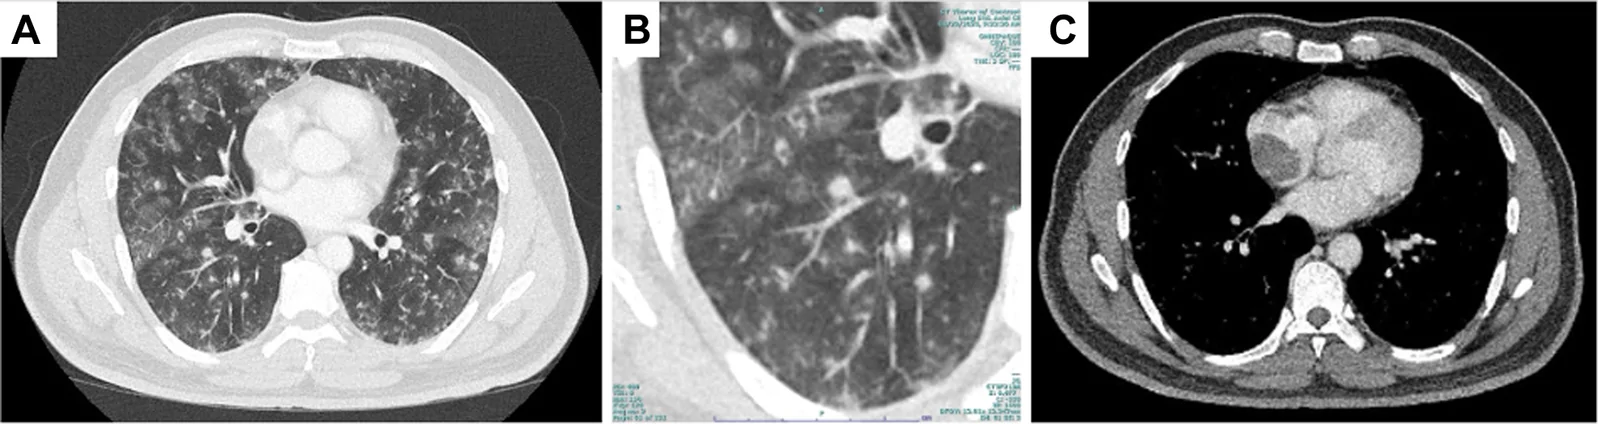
A, Chest CT scan in 3-mm slices revealed patchy ground-glass opacities with superimposed solid micronodules. Coned-down view of the right lower lobe showed solid nodules arranged in a tree-in-bud distribution. B, Most ground-glass opacities were centrilobular. C, Incidental finding of a right-sided atrial mass was seen in the soft tissue window.
